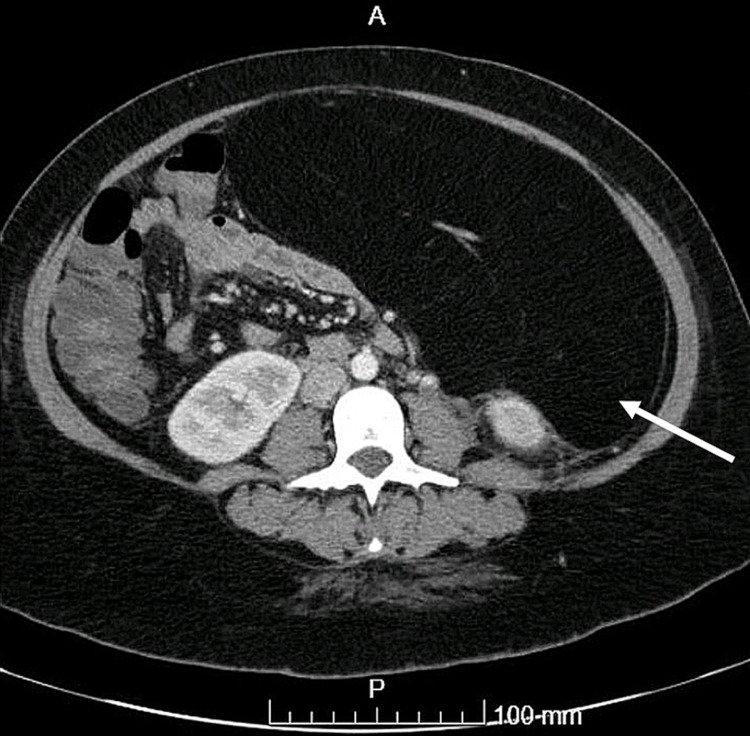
CT chest, abdomen, and pelvis for staging: large fat-containing lesion (arrow) seen intra-abdominally extending from the left upper quadrant.
